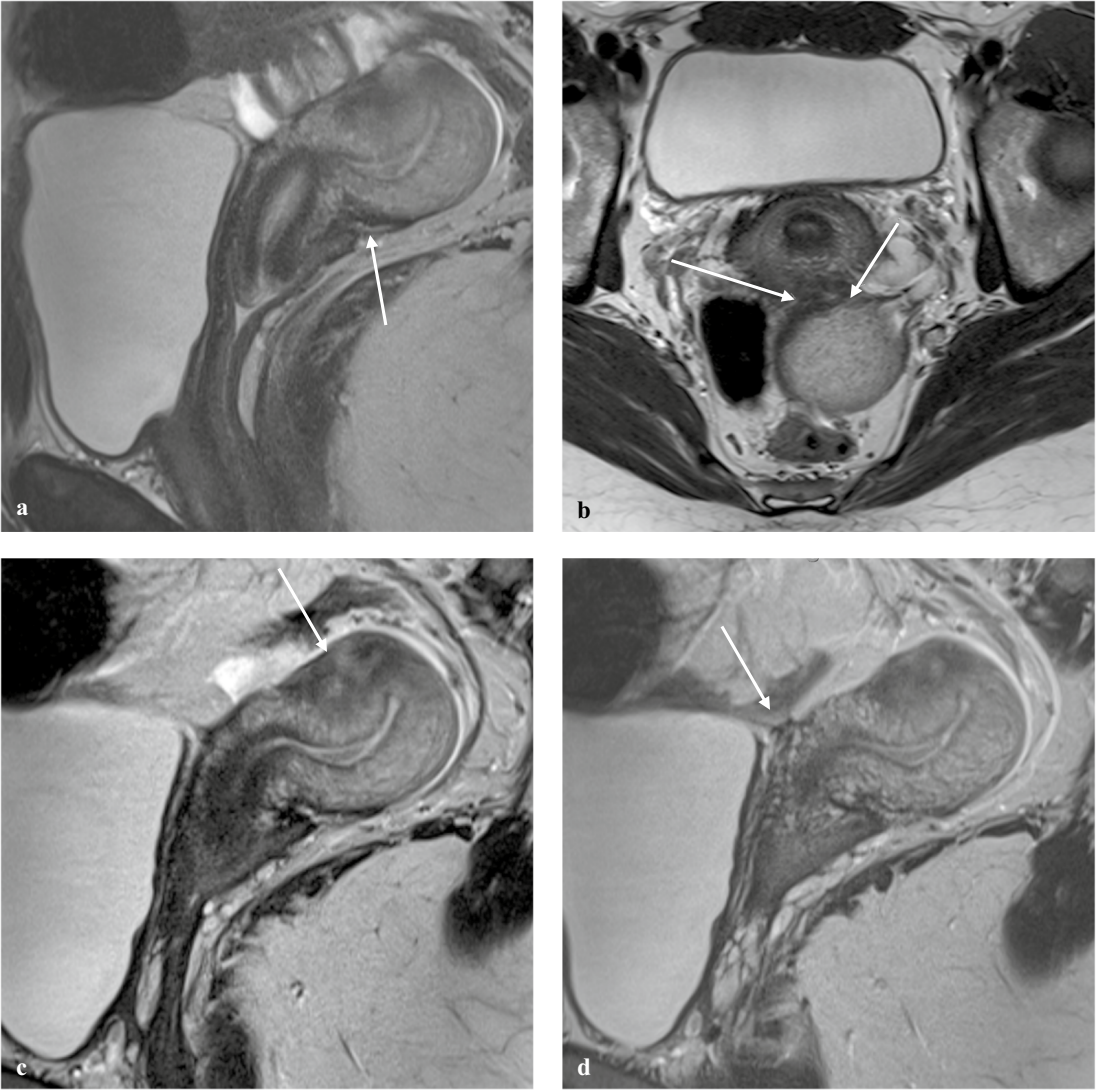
Example of the application of the #Enzian (m) classification, with MR images and coding. 22-year-old-patient with pelvic and small bowel endometriosis. a Sagittal T2W image shows a 0.5 cm endometriosis lesion in the rectovaginal space. b Axial T2W image demonstrates bilateral areas (approximately to 2 cm) of fibrotic thickening and low signal intensity in keeping with uterosacral ligamental involvement (arrows). c Sagittal T2W image shows focal adenomyosis on the anterior uterine wall (arrow). d Sagittal T2W image shows a focal thickening on the wall of a loop of ileum (arrow) in keeping with ileal endometriosis (arrow). Disease classification according to #Enzian (m): A1, B2/2, FA, FI
